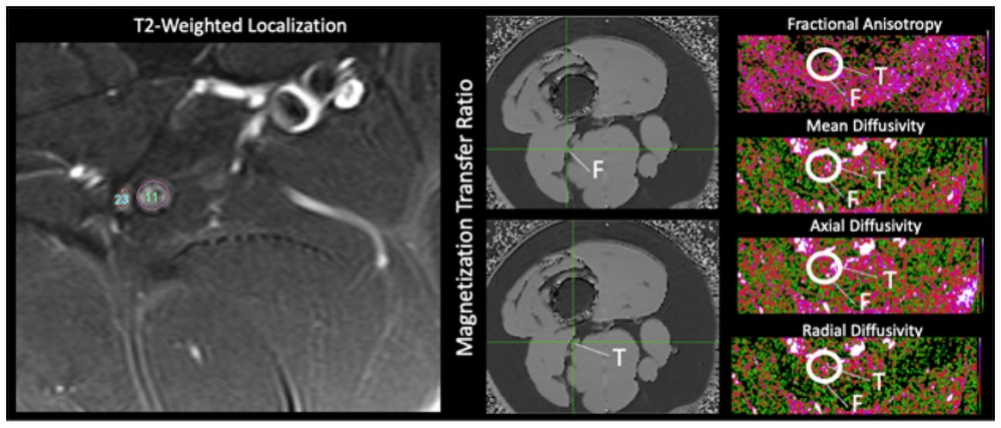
Regions of interest. Overview of how regions of interest were identified in the tibial and fibular nerve divisions using T2 Weighted Localization, and how the Tibial and Fibular nerves appeared on MTR and DWI sequences.
